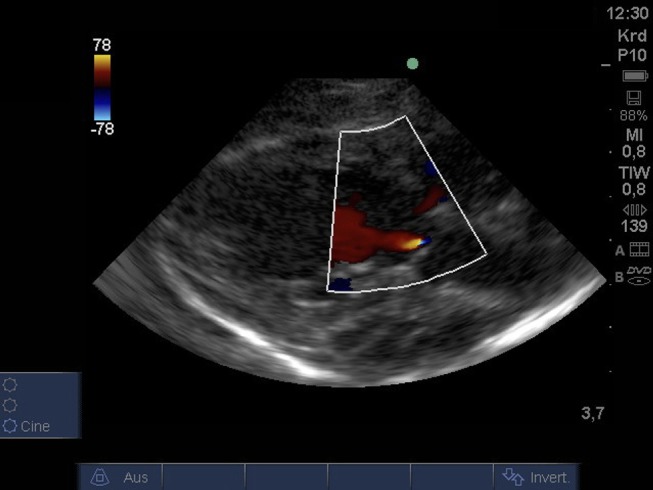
Color Doppler image of low-grade regurgitation across the aortic valve from PLAX (age: 36 h, weight: 1.48 kg). PLAX, parasternal long axis.
